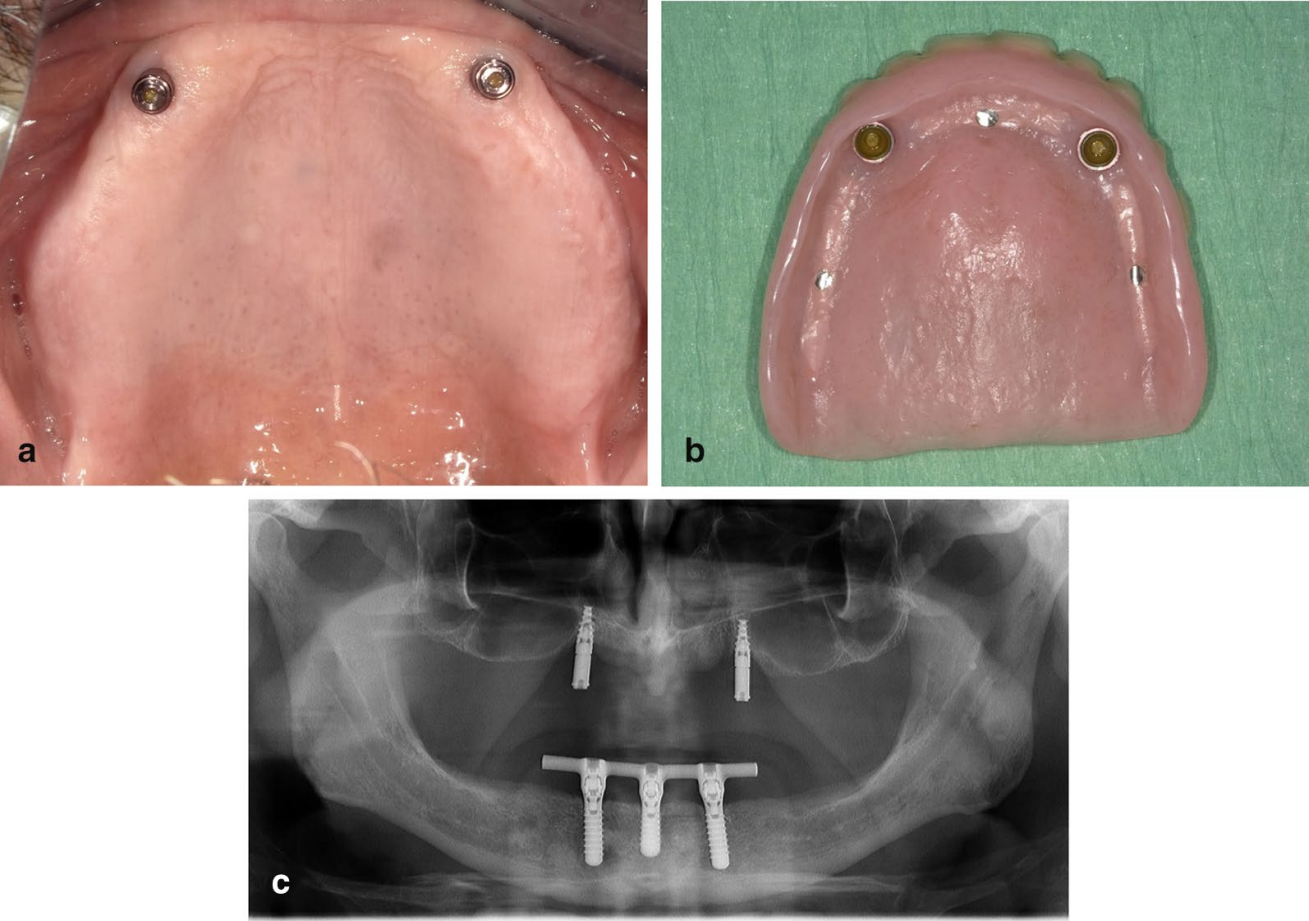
a Patient with a maxillary IOD after 12 months. b Overdenture of the same patient. c Panoramic radiograph of the same patient
